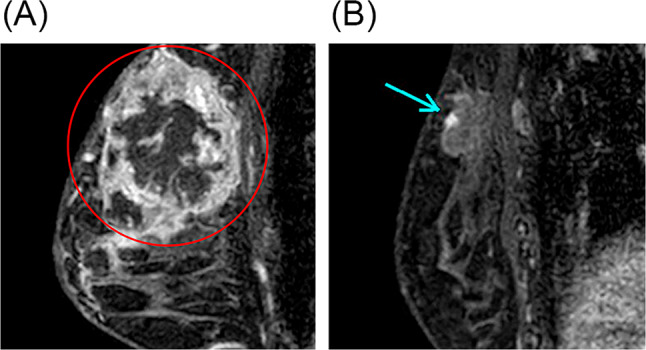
Contrast-enhanced magnetic resonance imaging of the right breast. (A) Before treatment. (B) After neoadjuvant chemotherapy
